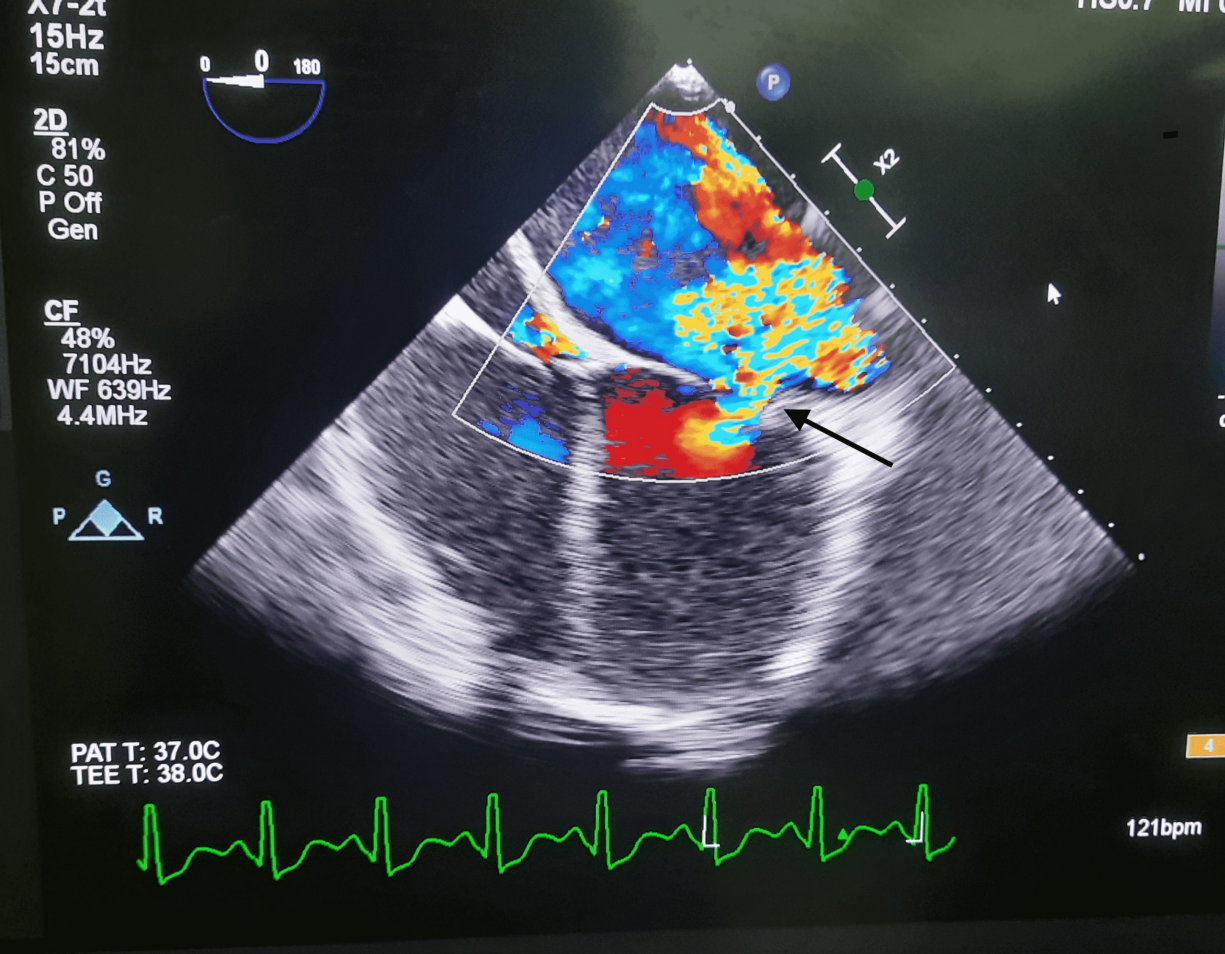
Severe mitral regurgitation with color jet viewed on transesophageal echocardiography
